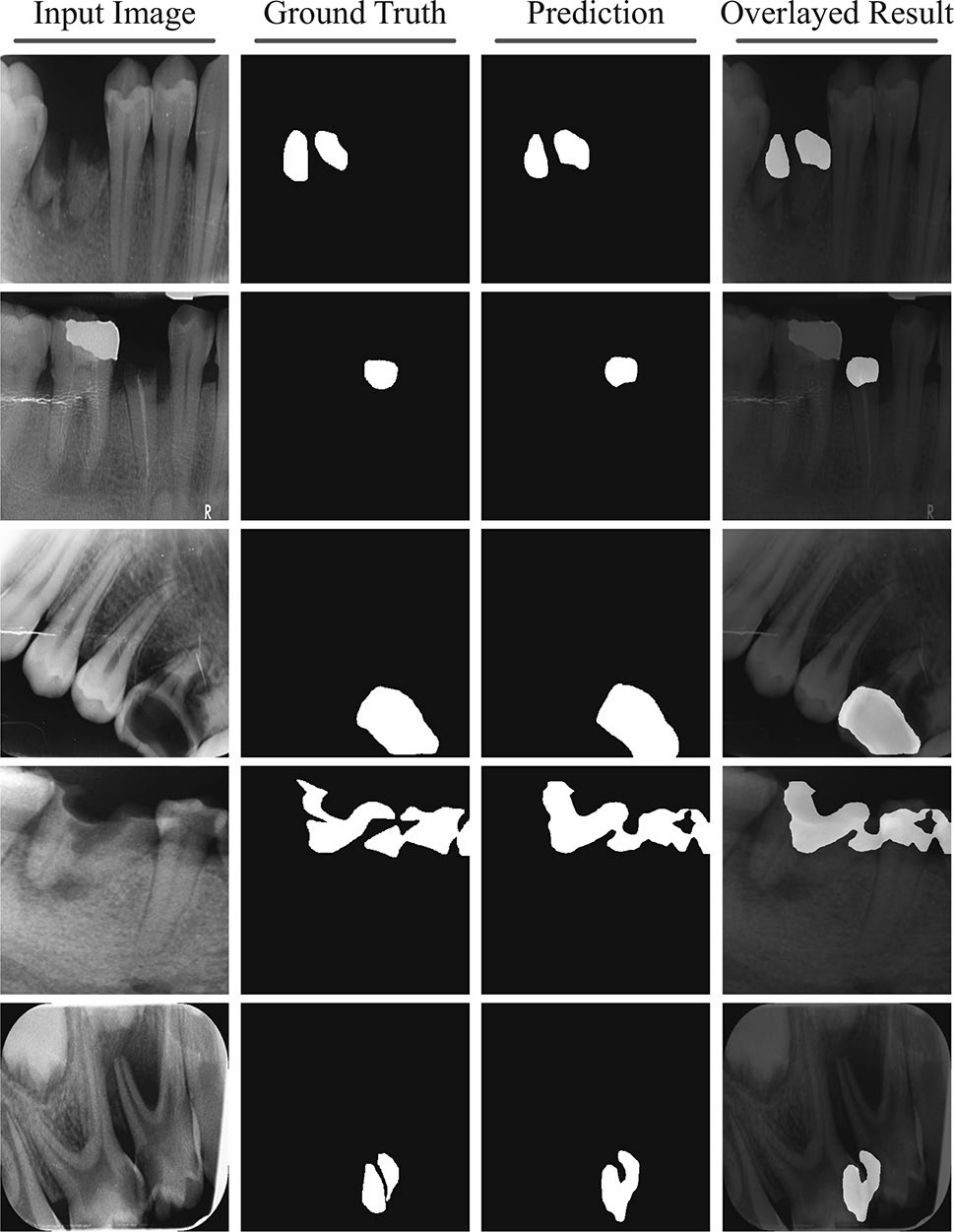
Qualitative results of proposed self-supervised learning strategy for caries detection in dental radiographs.
